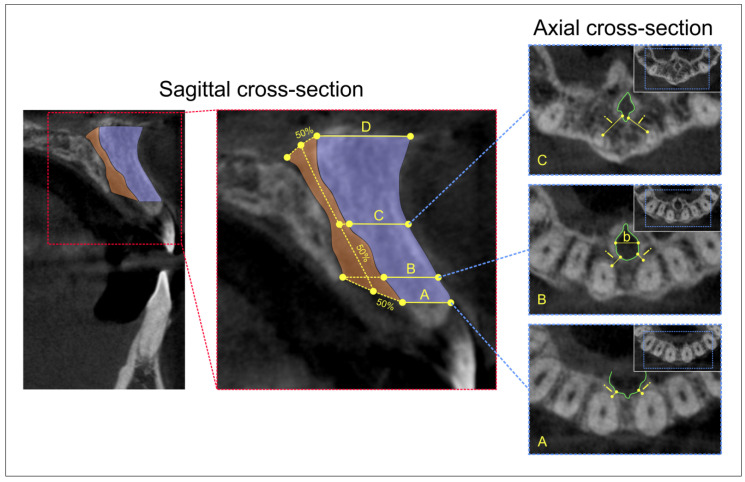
The definition of morphometric parameters of interest on CBCT images of the anterior maxilla. Sagittal cross-section; sagittal CBCT view with the marked field of interest (left); selected morphometric parameters for analyses (right): (A) the distance between the cortical layer of the incisive foramen and facial aspect of the buccal bone plate; (B) the distance between the cortical layer of the nasopalatine canal and facial aspect of the buccal bone plate using a horizontal line from the palatal border of the incisive foramen; (C) the distance between the cortical layer at the midpoint level of NPC length and facial aspect of the buccal bone plate; and (D) the distance between the cortical layer of the nasal foramen and facial aspect of the buccal bone plate. Axial cross-section; axial CBCT view: (bottom) the minimal interspace (i) between incisive foramen and central incisors at level A; (middle) the minimal interspace (i) between incisive foramen and central incisors at level B; (b) M-L diameter of incisive foramen; and (upper) the minimal interspace (i) between NPC and central incisors at level C.
